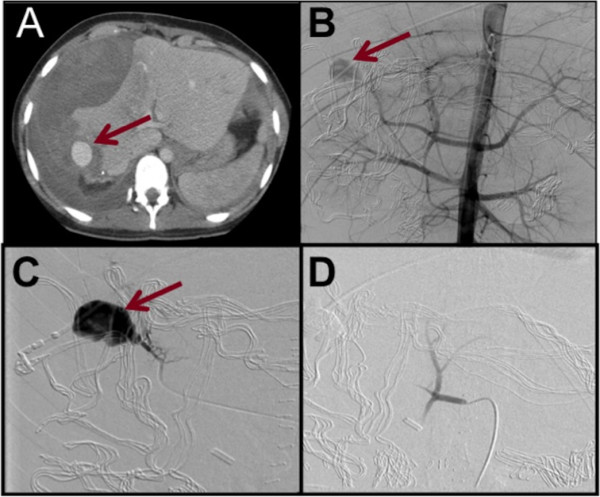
Right hepatic artery pseudo aneurysm. Arrows show the aneurysm on axial CT (A) and angiography (B). The pseudoaneurysm and feeding vessel (C) were coiled and no further filling was demonstrated (D).
